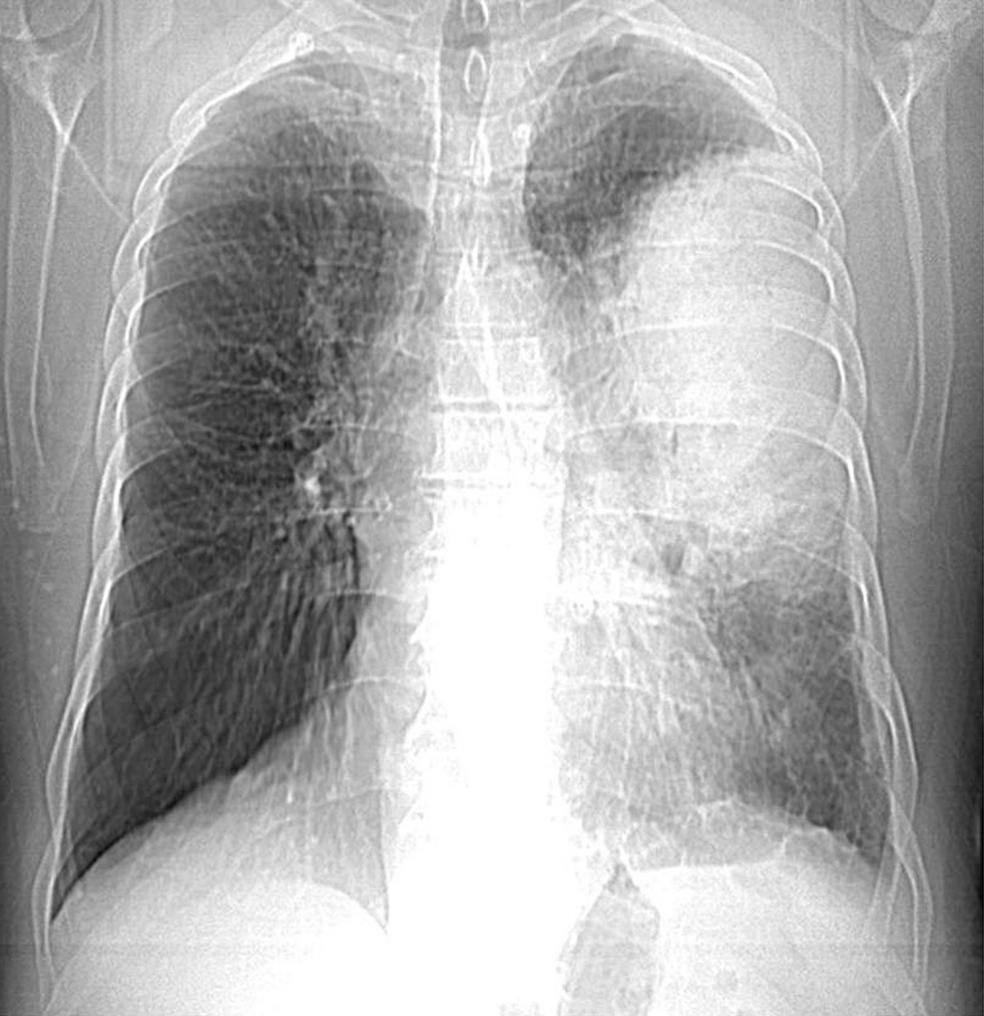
First chest X-ray showing alveolitis type shadowing taking up two-thirds of the left lung
